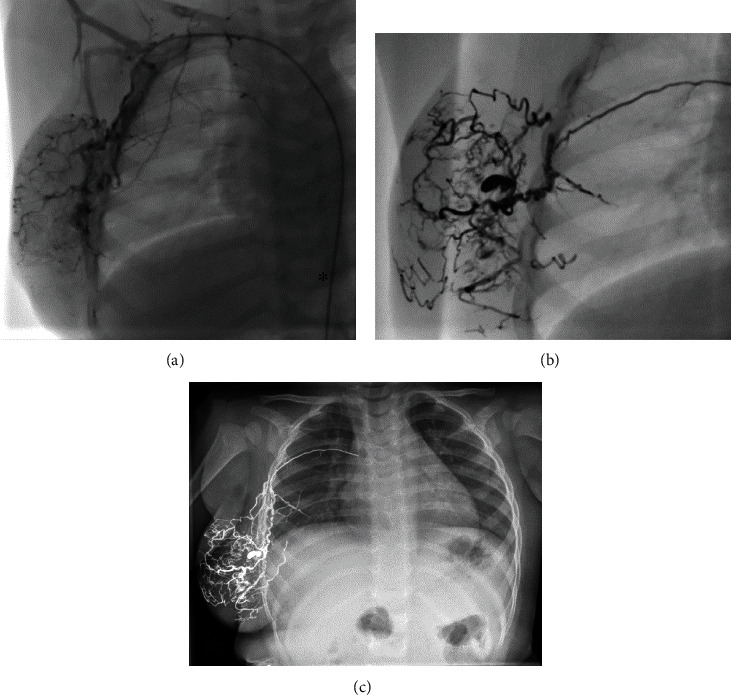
Imaging of the thoracic AVM in patient 2. (a) Angiographical imaging after injection of Solutrast 300® via a transarterial catheter (asterisk) into the feeding arteries deriving from the right subclavian artery. (b) Angiographical imaging after injection of radiopaque Onyx®. (c) Radiographic imaging (X-ray) of the thorax after the last embolization.
